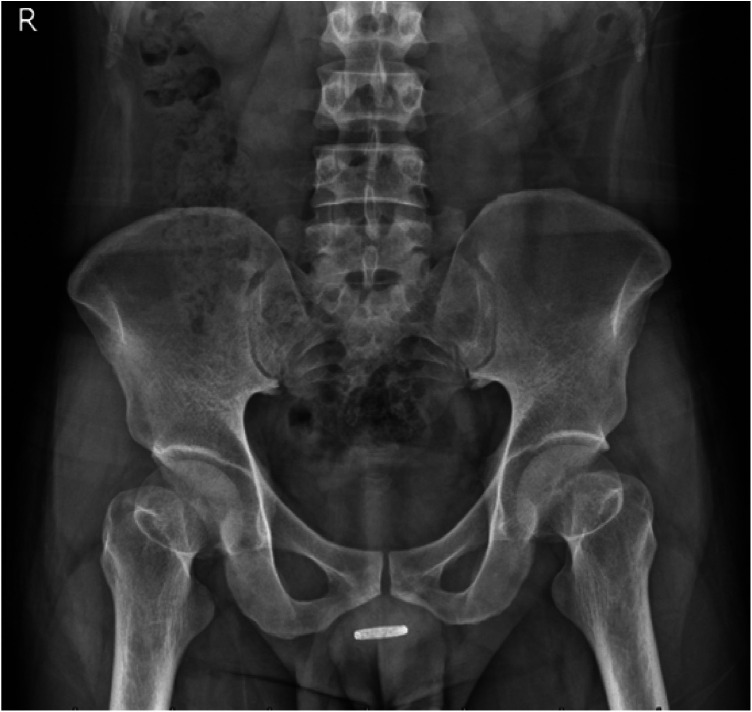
Plain x-ray examination confirms the presence of an embedded metal ring at the penile base.
